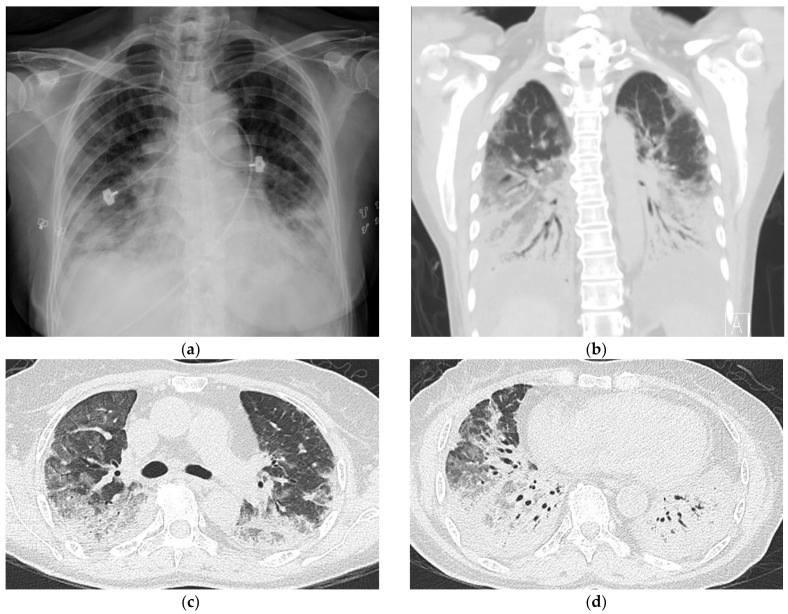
Chest imaging upon admission to the intensive care unit (a) Chest radiograph revealed new development of bibasilar consolidations. (b–d) Computed tomography scans revealed consolidations and increased ground-glass opacities over the bilateral lungs.
